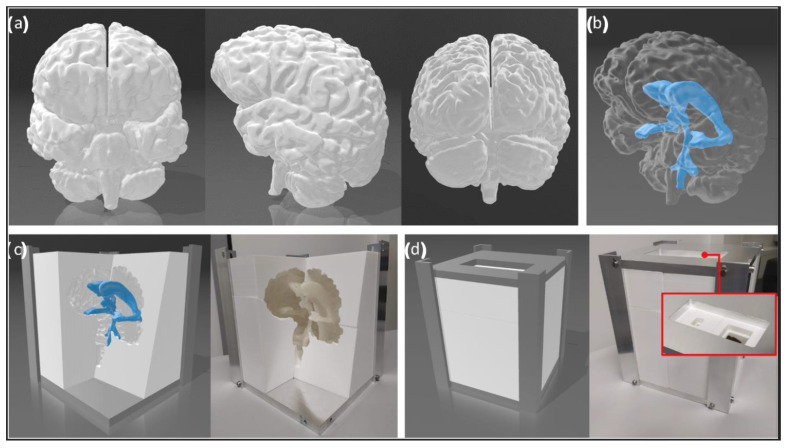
(a) Computational model of the brain, segmented from the MR image. (b) Computational model of the ventricles, located inside the brain. (c) Inside view of the computational model of the brain mold and corresponding inside view of the 3D-printed brain mold. (d) Outside view of the computational model of the brain mold and corresponding outside of the 3D-printed brain mold. The red frame shows the large and small openings at the top.
